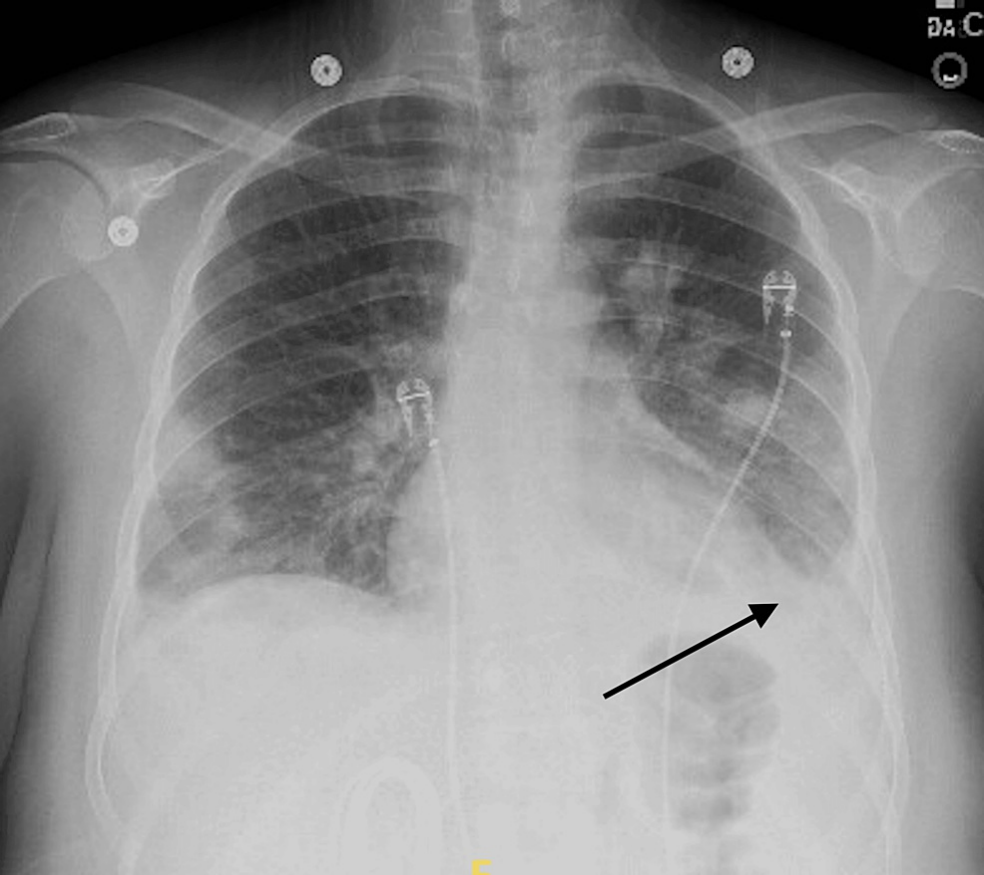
Chest radiography with small left pleural effusion and consolidation
